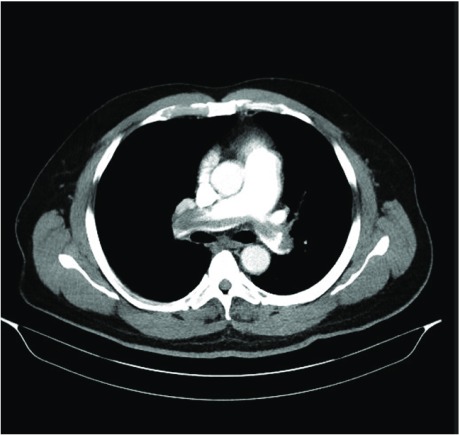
Axial CTA of the chest showing a saddle embolus with extension into the branches of the pulmonary artery. CTA was performed using Siemens SOMATOM Perspective 128 slices. Images were obtained in a cranio-caudal direction following contrast injection at 3mls/s. Contrast optimization was based on bolus tracking at the level of the main pulmonary artery using a trigger level of 100 HU.
